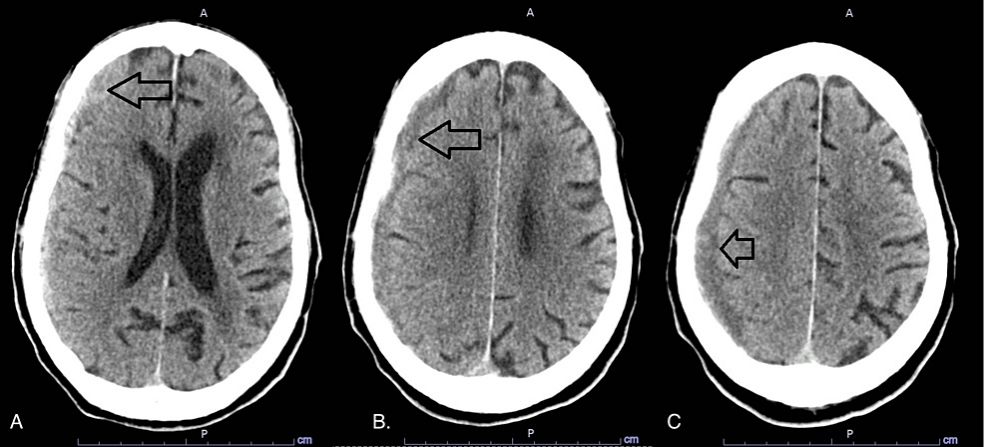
CT scan of the head Computed tomography (CT) scan of the head shows right-sided subdural hemorrhages with different densities at different levels (arrows in A, B, and C)
